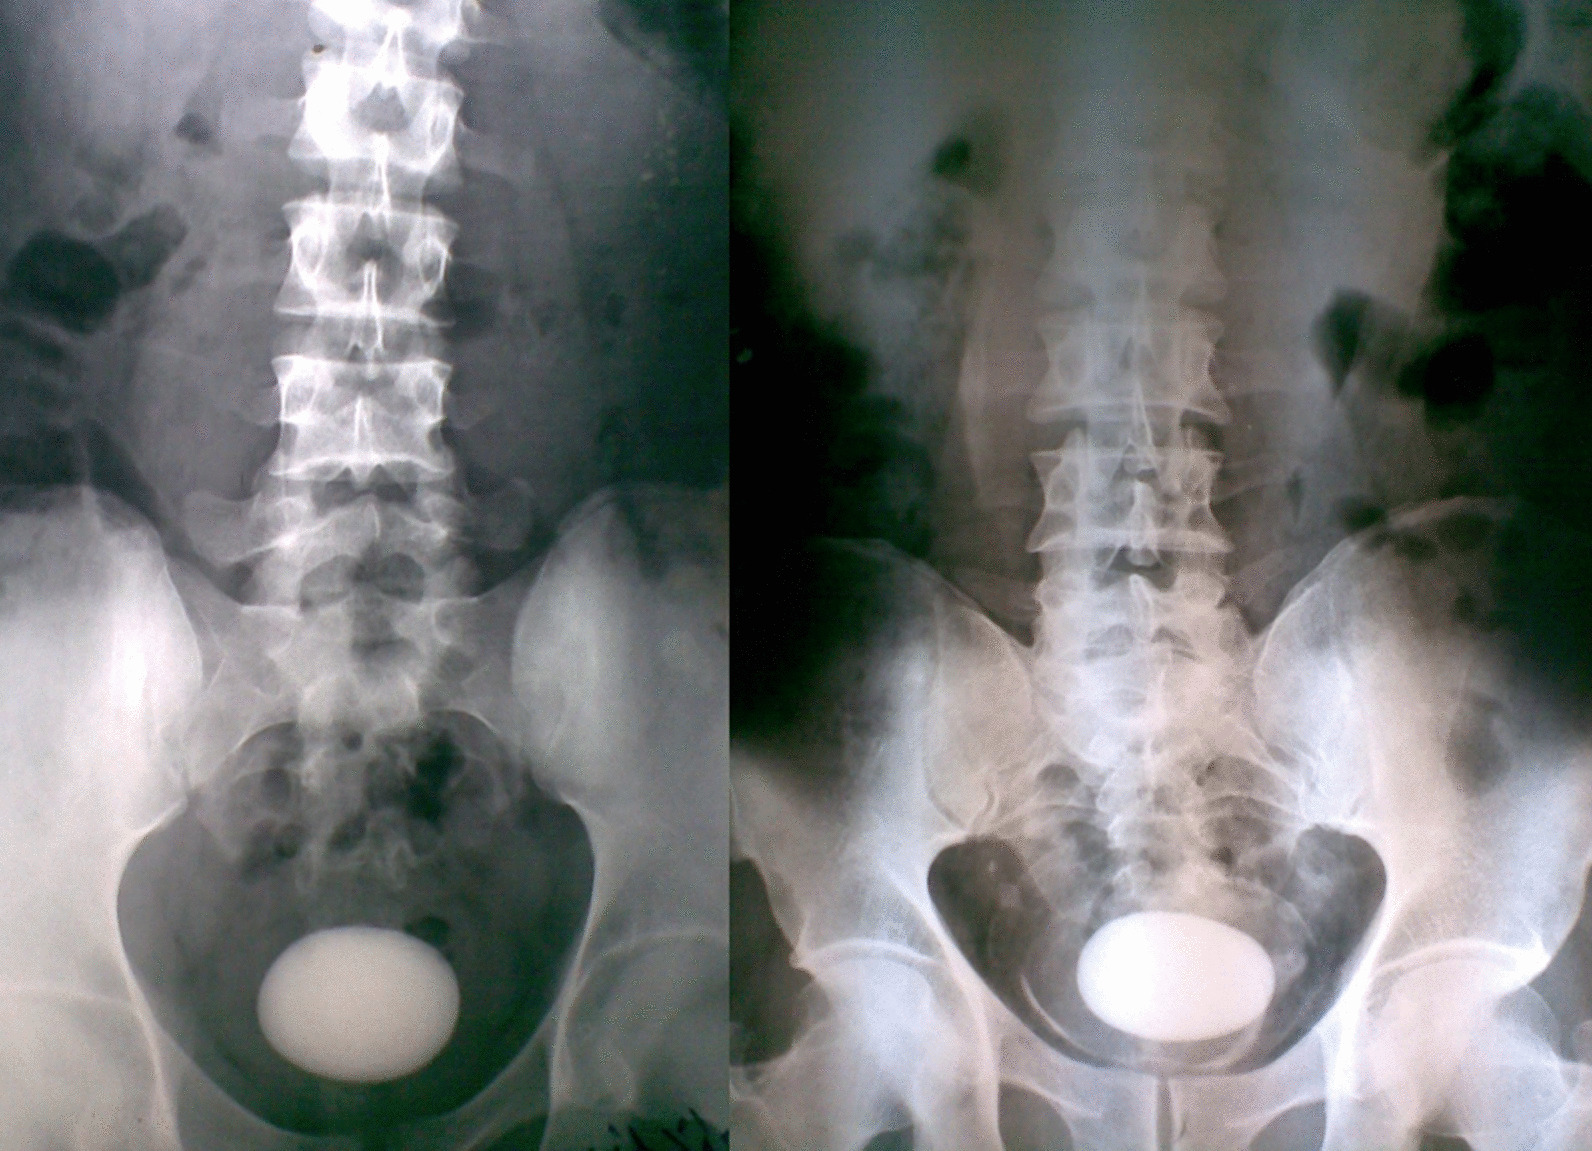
Giant stones of the urinary bladder with oval shapes, smooth surfaces, and high densities in plain radiographs. Notice the linear bilharzial calcifications of the urinary bladder in the right-sided figure
